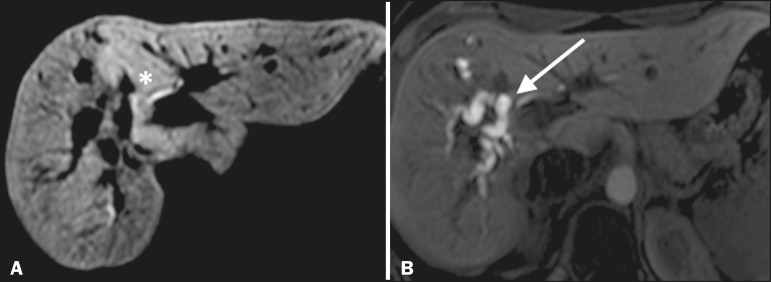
Stenosis of the intrahepatic biliary tract after cholecystectomy. Axial T1-weighted fMRC sequences acquired at 20 min (A) and 4 h (B) after the injection of gadoxetic acid, showing moderate dilatation of the right biliary tract caused by stenosis in the plane of the right bile duct (arrow in B). Note that the concentration of contrast medium is higher in the parenchyma of the left hepatic lobe (asterisk in A) than in that of the right lobe, where there is a delay in the enhancement of the bile ducts (B). Diagnoses of thermal injury and bile duct ligation were considered.
